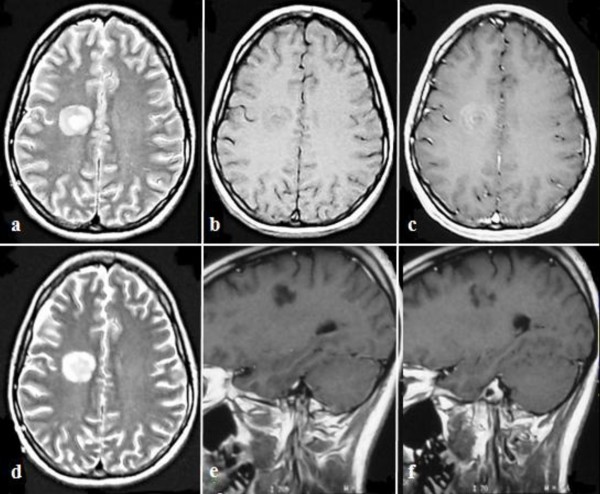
Brain MR images in a 20-year-old woman. (a) Axial T_2_-weighted image shows a hyperintense mass with concentric pattern in the right centrum semiovale. (b) Axial T_1_-weighted image reveals hypoisointense concentric rings in the white matter of the right parietal lobe. (c) Axial T_1_-weighted image after contrast shows concentric enhancing rings. (d) Axial T_2_-weighted image, 1 month after therapy, shows differences with decrease of the signal intensity at the center of the lesion. (e, f) Coronal T_1_-weighted image after administration of gadolinium demonstrates a low-signal, non-enhancing lesion.
